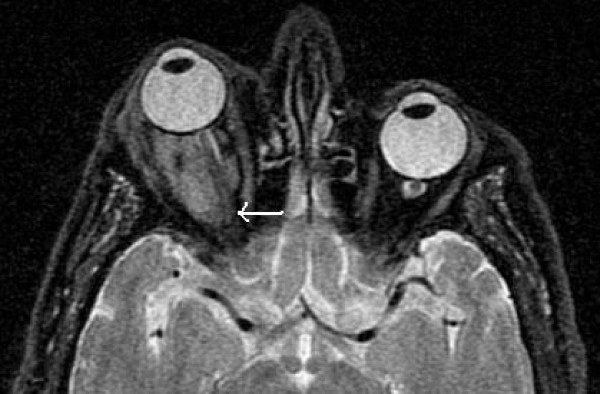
Axial MR image (T2 with fat saturation). The lesion is slightly hyperintense to the optic nerve (arrow).
